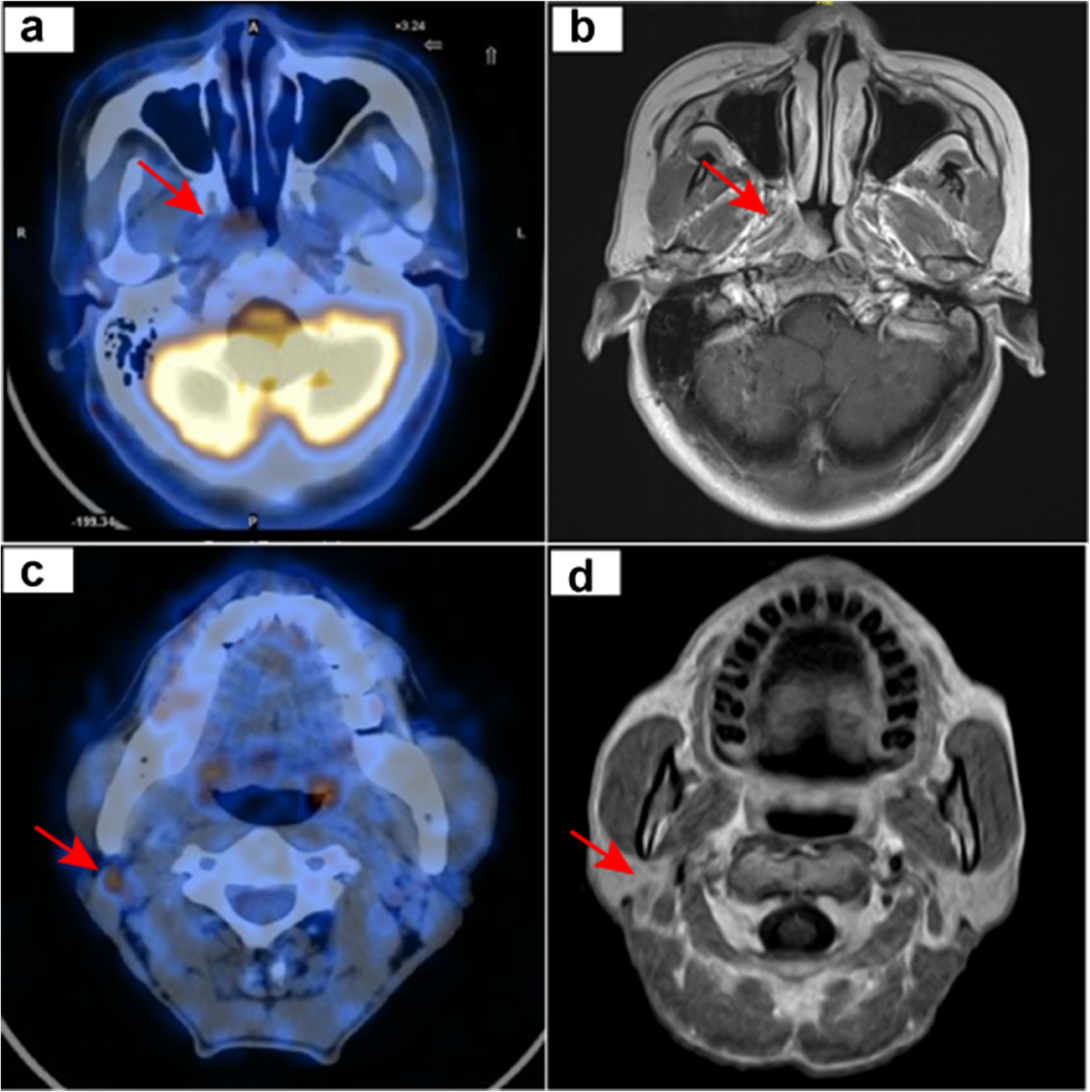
T- and N-staging discrepancies between PET/CT and head-and-neck MRI. (a, b) Female, 59 years old, T2N0M0 NPC. PET/CT image (left). Contrast-enhanced T1-weighted MRI image (right). PET/CT did not detect the parapharyngeal lesion that was found by MRI (red arrow). (c, d) Male, 57 years old, T2N1M0 NPC. PET/CT image (left). Contrast-enhanced T1-weighted MRI image (right). PET/CT distinguished positive neck lymph node (red arrow) while it was regarded as a morphological benign lymph node in MRI
